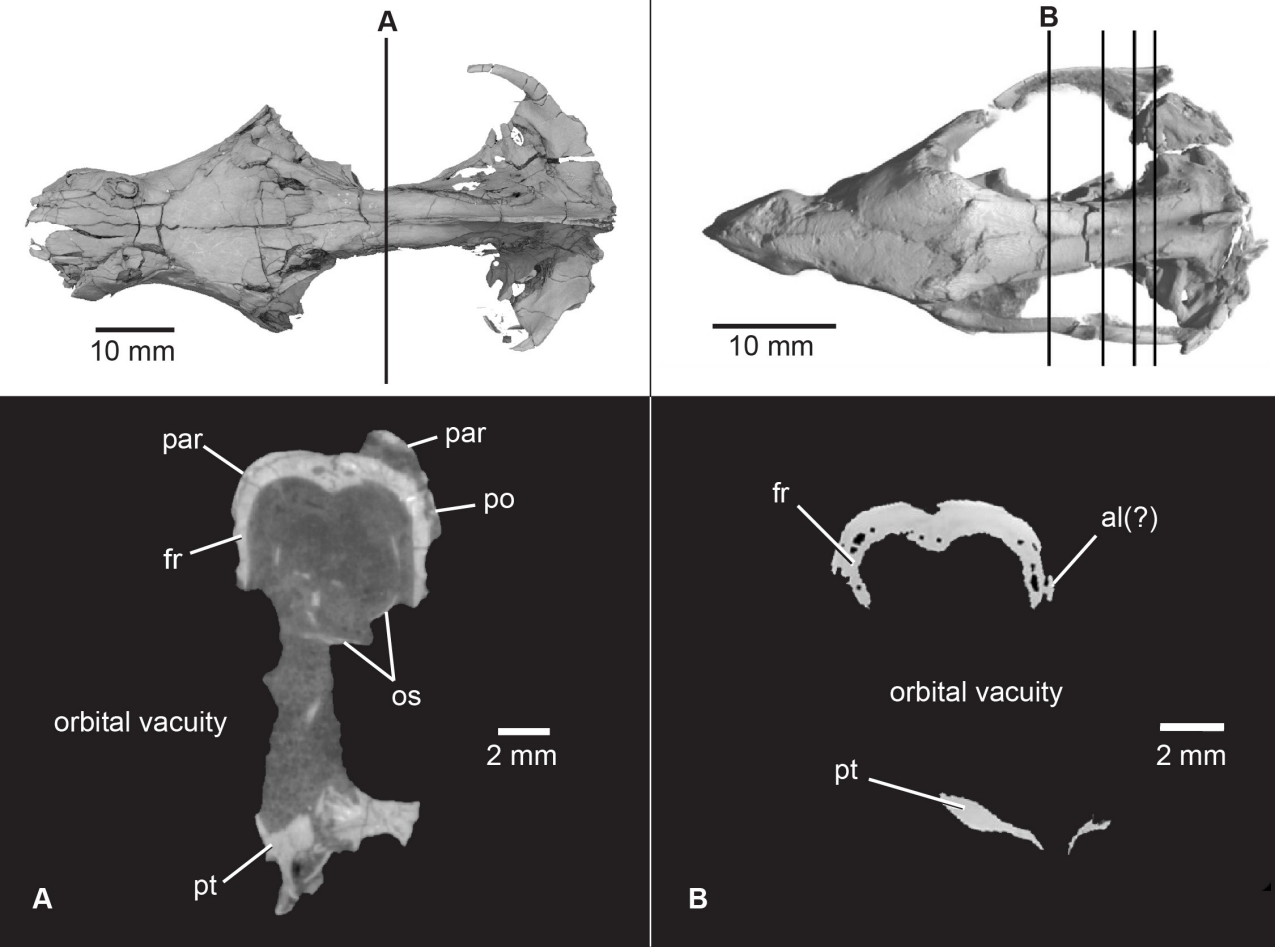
Comparison of orbital vacuities in *Pseudotherium* and *Brasilitherium riograndensis*. The ventral limit of the endocranium in *Pseudotherium* (A) is dorsal to the orbital vacuity and lined by ossified orbitosphenoids. The orbitosphenoids are missing in *Brasilitherium* (B) but were likely present, suggesting a shallower endocast than previously hypothesized. *Brasilitherium riograndensis* CT images from Rodrigues et al., ([60]: Fig 3). Abbreviations: al, alisphenoid; fr, frontal; os, orbitosphenoid; par, parietal; po, postorbital; pt, pterygoid.
